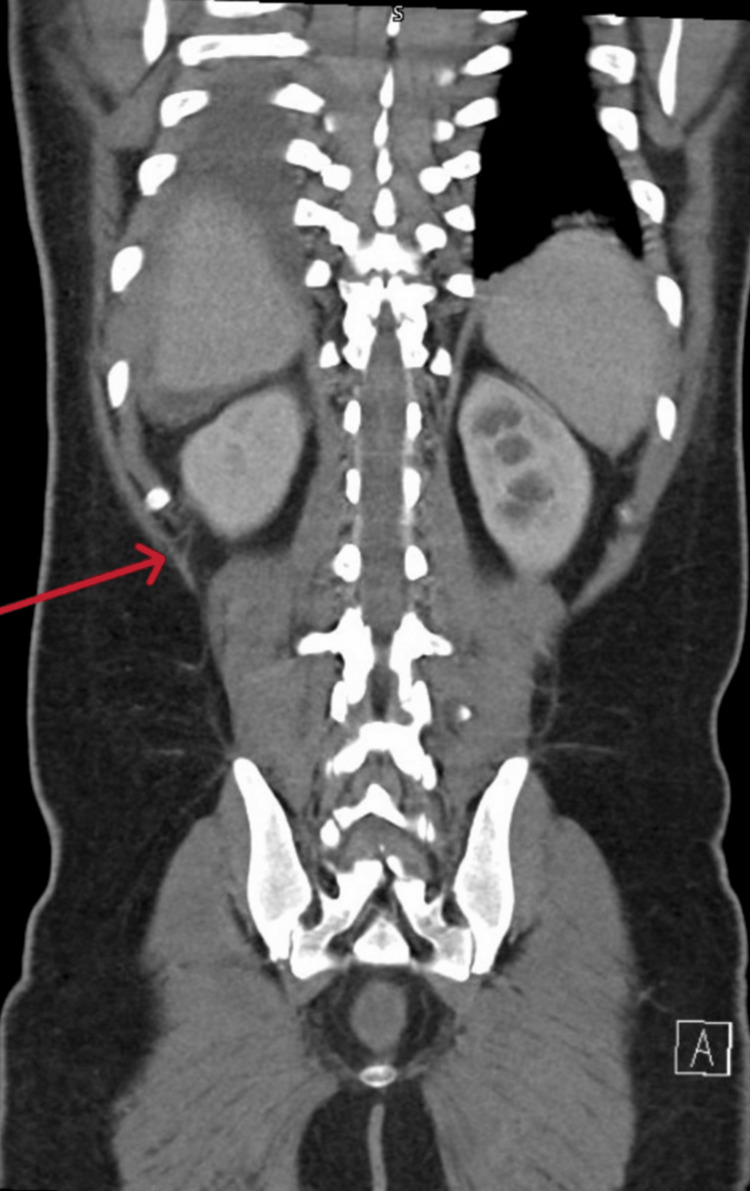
CT scan/coronal view revealed pocket of fluid collection in the right pelvic area with enhancing wall (red arrow)
